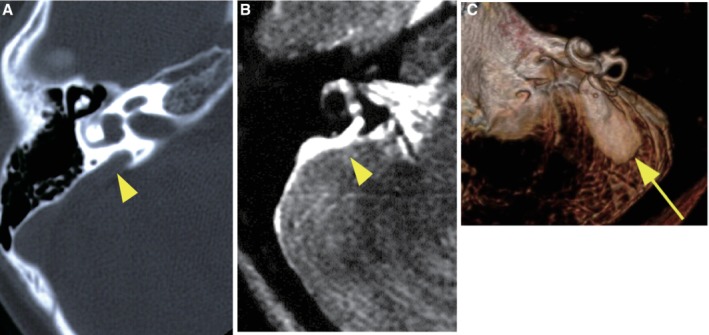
A: Computed tomography (CT) scan of an enlarged vestibular aqueduct (arrowhead), B: Magnetic resonance (MR) image of an enlarged endolymphatic duct (arrowhead), C: 3D imaging of an MR image of an enlarged endolymphatic duct and sac (arrow).
